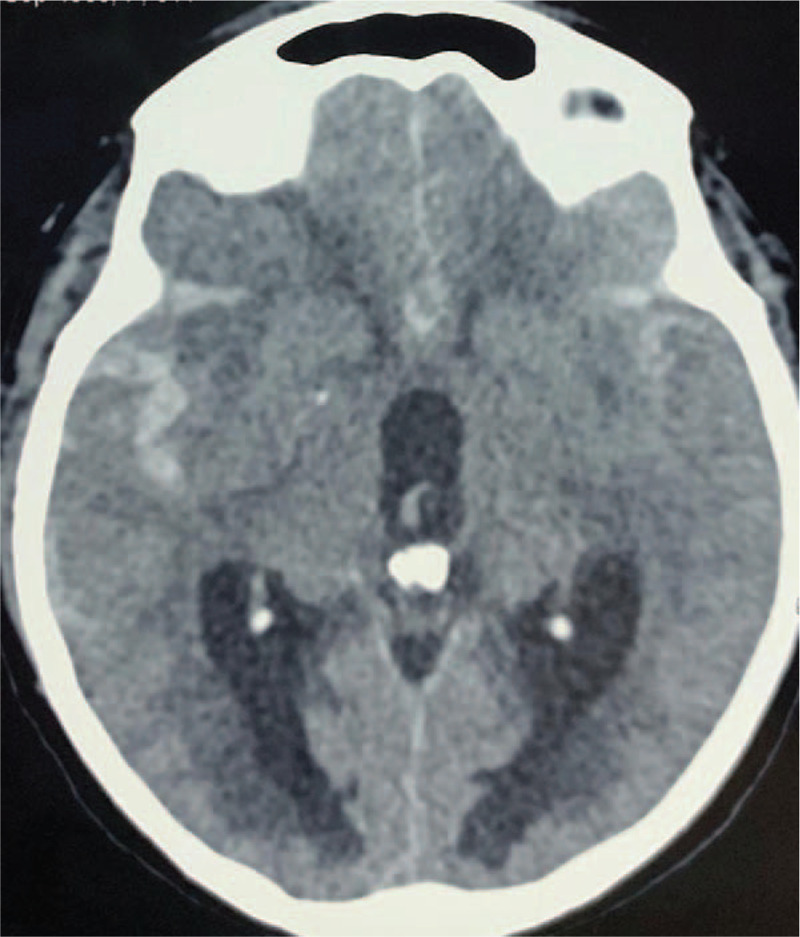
Cranial CT scan (axial slice) shows foci of SAH in both Sylvian fissures (more marked on the right side) and acute hydrocephalus.
